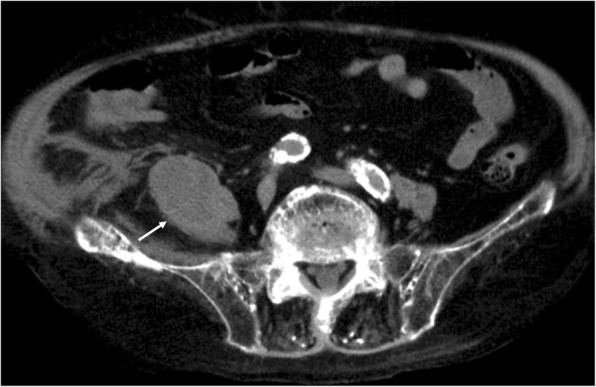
Abdominal computed tomography on admission showed a low-density mass in the right iliopsoas muscle (*white arrow*)
